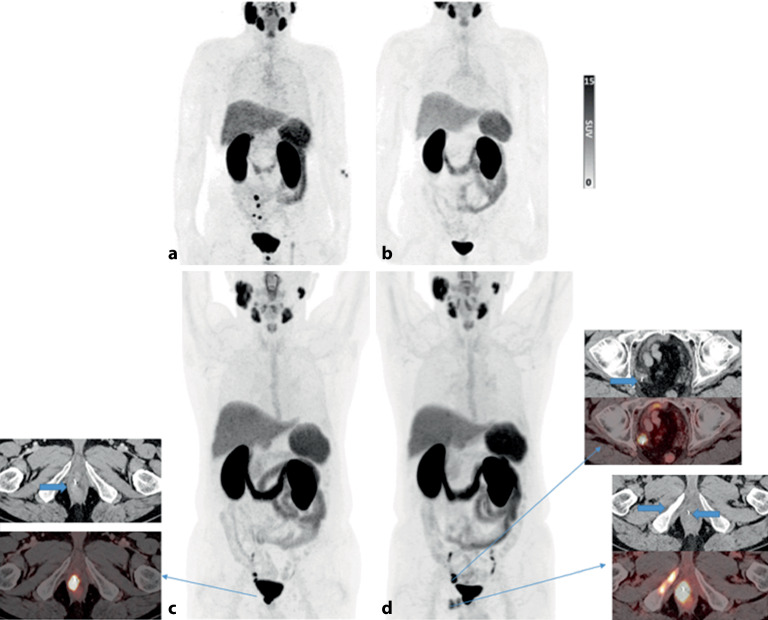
[^68^Ga]Ga-PSMA-11 PET skull base to thigh scans throughout multiple cycles of [^177^Lu]Lu-PSMA RLT. a [^68^Ga]Ga-PSMA-11 PET imaging demonstrates PSMA-avid lymph node metastases and local recurrence after radical prostatectomy in the pelvic region before therapy initiated in March 2016; b PSMA-PET showing a reduction in volume and avidity of multiple PSMA lymph nodes in the retroperitoneal and right parailiac regions after three PSMA-RLT cycles in August 2016; c [^68^Ga]Ga-PSMA-11 PET/CT imaging before the second rechallenge with progressive local recurrence and enhanced PSMA uptake in retroperitoneal lymph nodes (blue arrows); d PSMA-PET/CT after completion of 12 RLT cycles demonstrates progression in right parailiacal lymph nodes, further progression of local recurrence in prostate bed, and a new lesion in the right pubic bone, marked by blue arrows.
